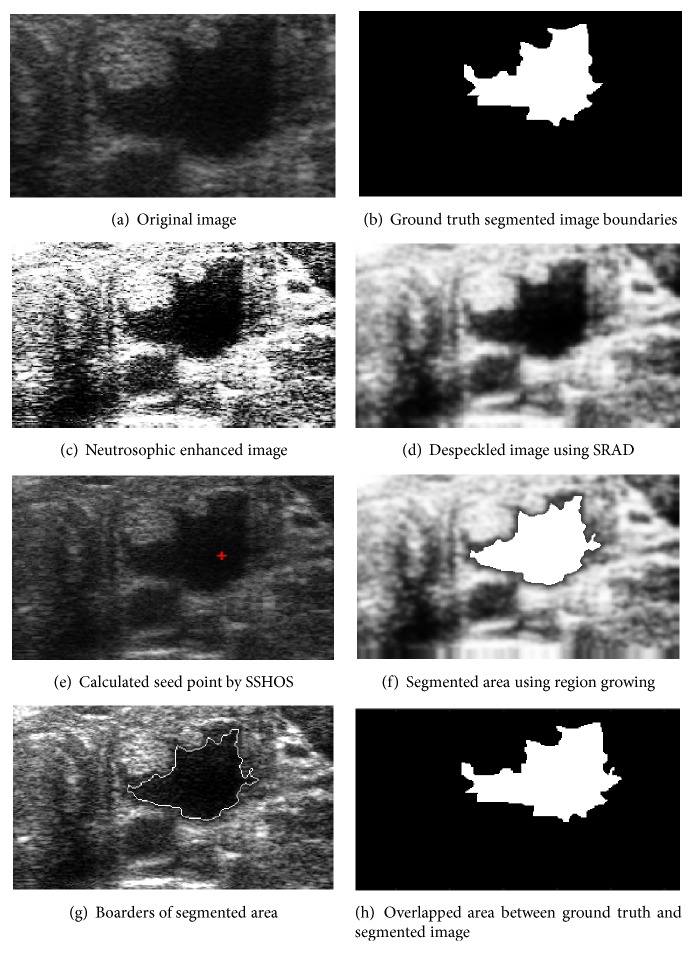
Ultrasound image (image 12 M).
